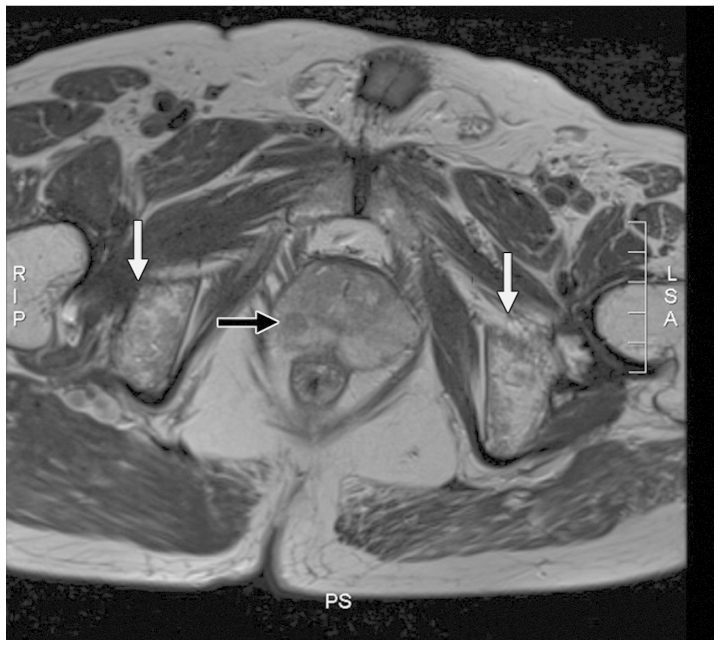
Magnetic resonance imaging of the prostate revealing a small nodule with abnormal signals located in the right prostatic peripheral zone (black arrow). The prostate capsule was continuous and complete, without lymph node enlargement. The signal intensity of the pelvic bone marrow was diffusely inhomogeneous (white arrows), and the structure of the cortical bone was intact.
